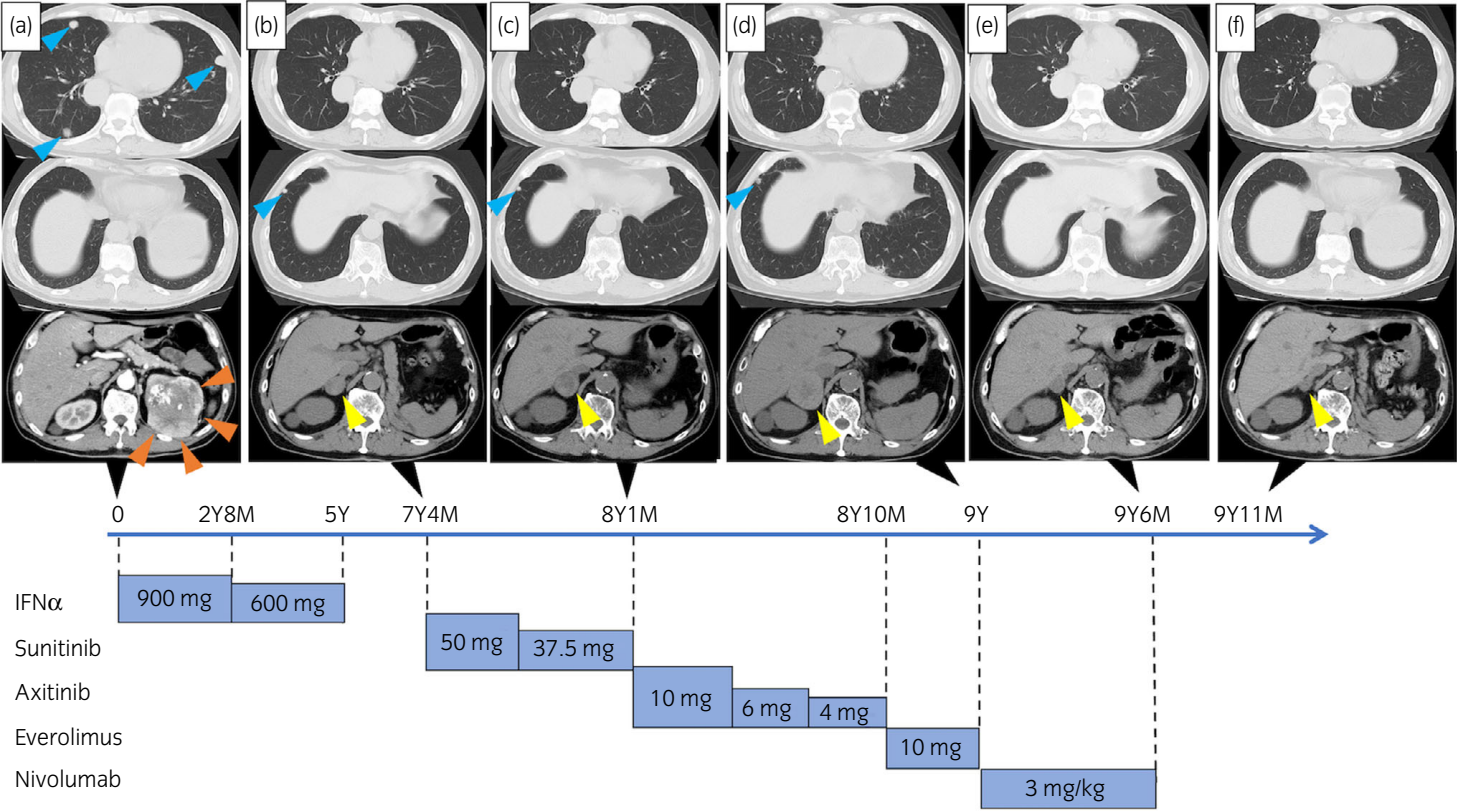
Clinical course of the case. CT images of representative lesions of the renal tumor (orange arrowheads), lung metastasis (blue arrowheads), and right adrenal gland metastasis (yellow arrowheads). (a) CT scan at primary diagnosis. (b) CT revealed new lung and right adrenal gland metastasis. (c) CT revealed lung metastasis shrinkage but adrenal gland metastasis growth. (d) CT revealed adrenal gland metastasis growth. (e) CT revealed adrenal gland metastasis remarkably reduced in size by nivolumab. (f) Five months after discontinuing nivolumab, CT revealed that adrenal gland metastasis continued to shrink. IFNα was administered weekly, each of the TKIs daily, and nivolumab once every 2 weeks.
